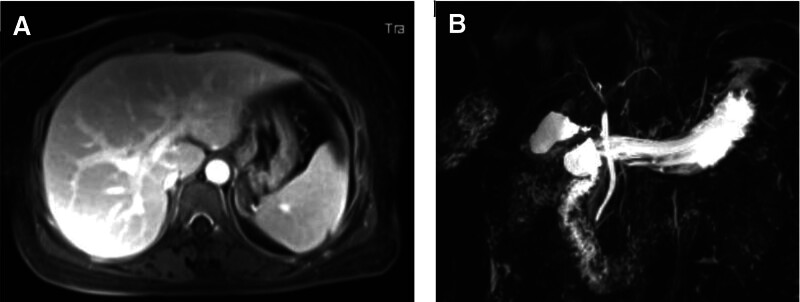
Magnetic resonance imaging (A) and magnetic resonance cholangiography (B).
